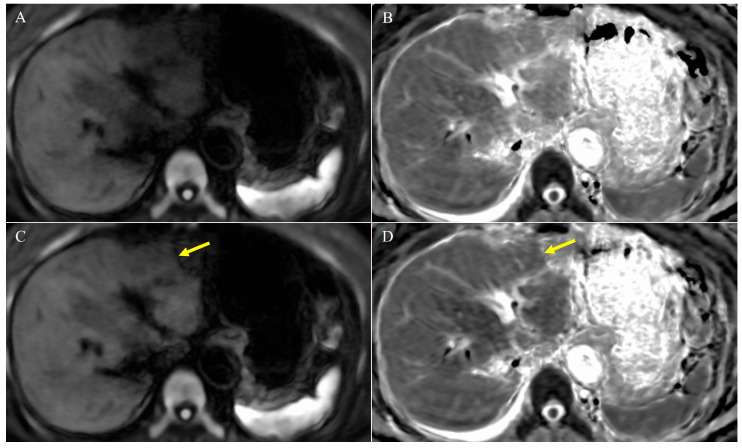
Comparison of BH non-ACSA (conventional) DWI (A) and corresponding ADC map (B) and BH-ACSA DWI (C) and corresponding ADC map (D). On BH-ACSA DWI, the signal intensity of the liver parenchyma in the left lateral segment showed a distinct signal increase (arrow in (C)) compared to BH non-ACSA DWI, with signal intensity comparable to that of the right lobe. In the BH-ACSA ADC map, the overestimation of ADC values observed in the lateral segment on the BH non-ACSA ADC map is improved, and the inter-lobar difference in ADC values is scarcely observed (arrow in (D)).
